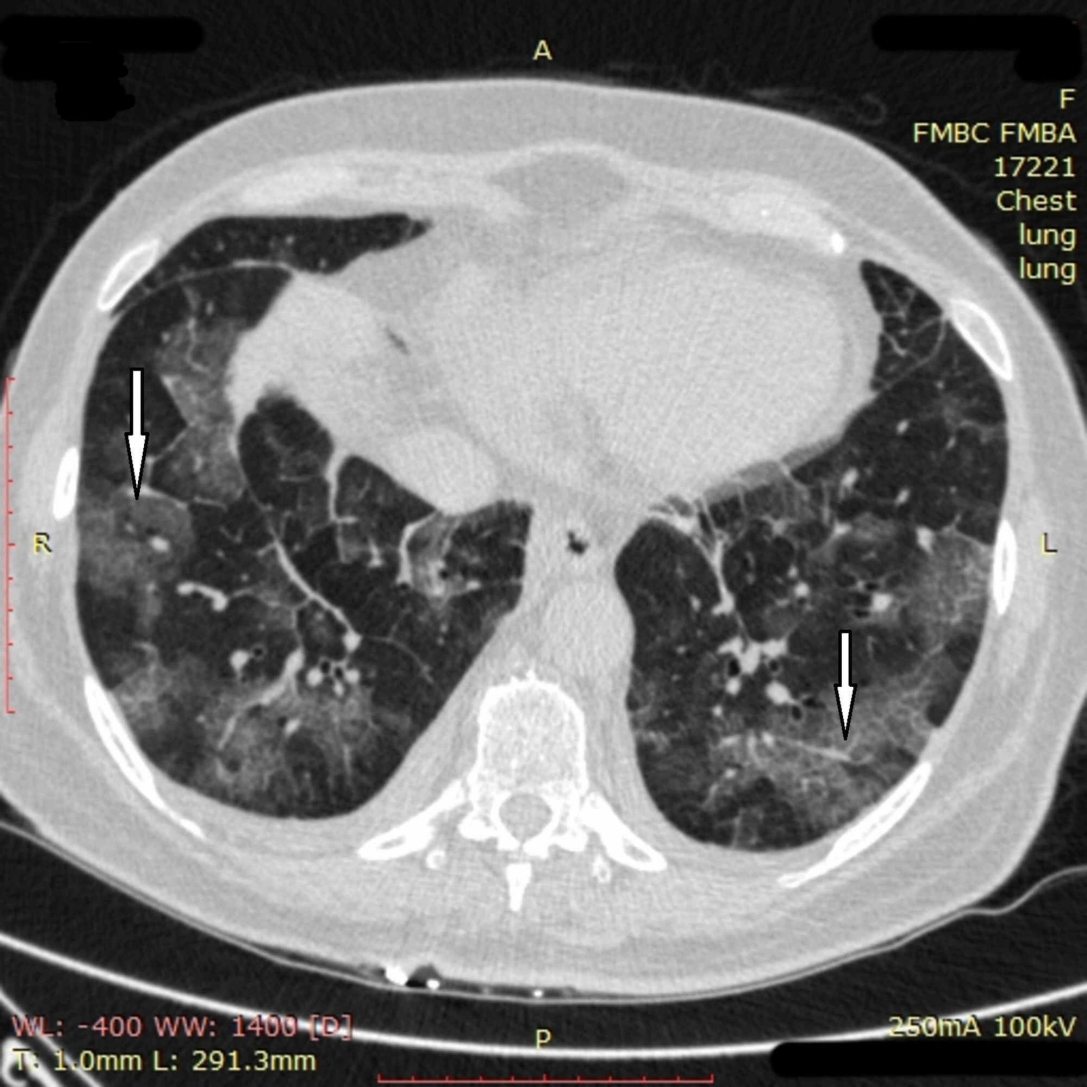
Chest CT imaging of patient 2 on day eight CT scan on day eight revealed an increase of pre-existing opacities in size and density. New ground-glass opacities appeared in upper and lower zones of the lungs merging into large subpleural opacification areas (white arrows). In the left lung, small foci of consolidation were seen. Interlobular septal thickening could be noted in various parts of the lungs. Overall, pathological changes involved at least 75% of pulmonary tissue CT: computed tomography
